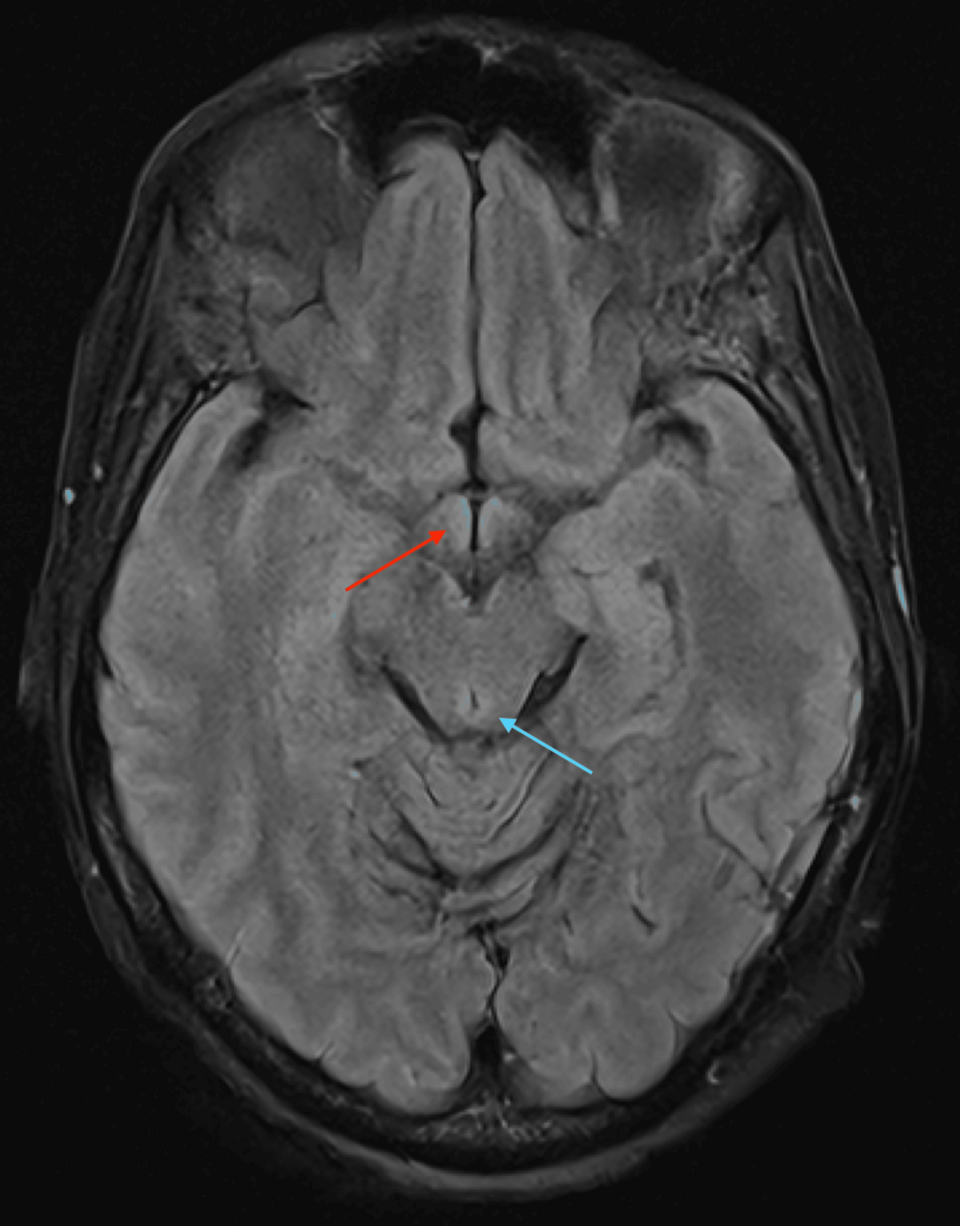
Subtle T2 FLAIR signal abnormalities, which may reflect developing magnetic resonance imaging findings. The red arrow indicates subtle hyperintensity in the mammillary bodies. The blue arrow indicates subtle hyperintensity in the periaqueductal gray matter. FLAIR: fluid-attenuated inversion recovery.
